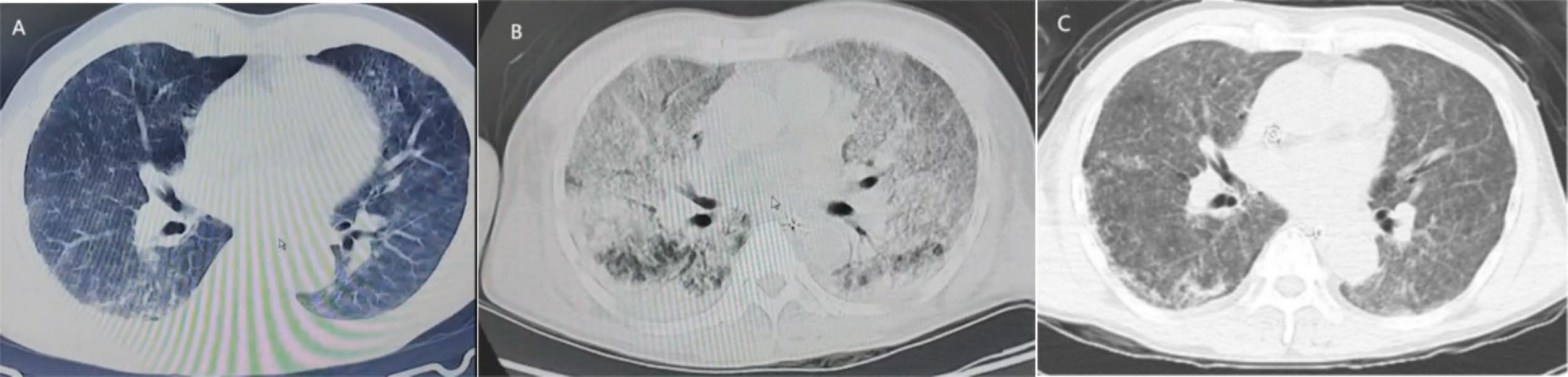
The image shows the patient’s lung condition and changes after treatment. (A) On June 11, the patient’s lung CT showed slight exudative lesions in both lungs. (B) On June 13, pulmonary CT showed diffuse exudative lesions in both lungs. (C) On June 17, lung CT showed significant improvement after treatment.
